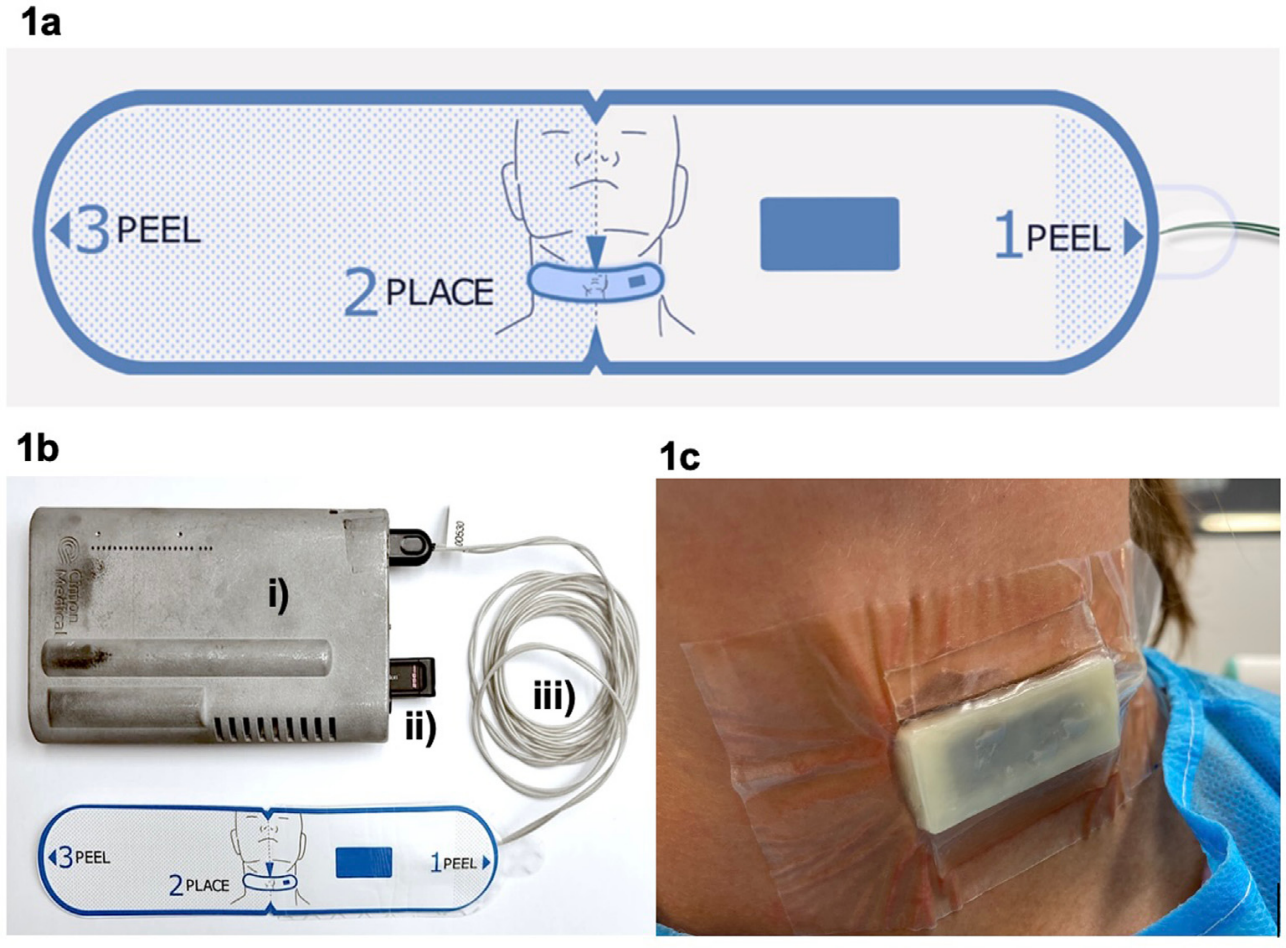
(a) The RescueDoppler application guide. (b) The RescueDoppler system: (i) host unit with ultrasound scanner and computer, (ii) USB drive, (iii) extension cable. (c) The RescueDoppler probe and self-adhesive patch are shown in place, following the removal of the application guide.
